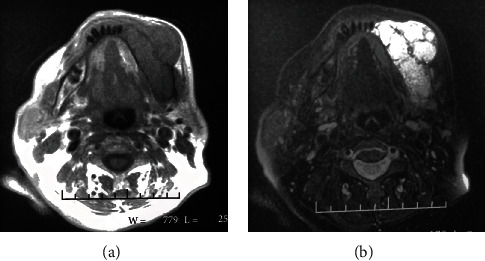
10-year MRI images: (a) after an axial T1WI MR image showing the stabilization of the old lesion; (b) an axial T2WI image presented the same hypersignals and expansible lesion.
